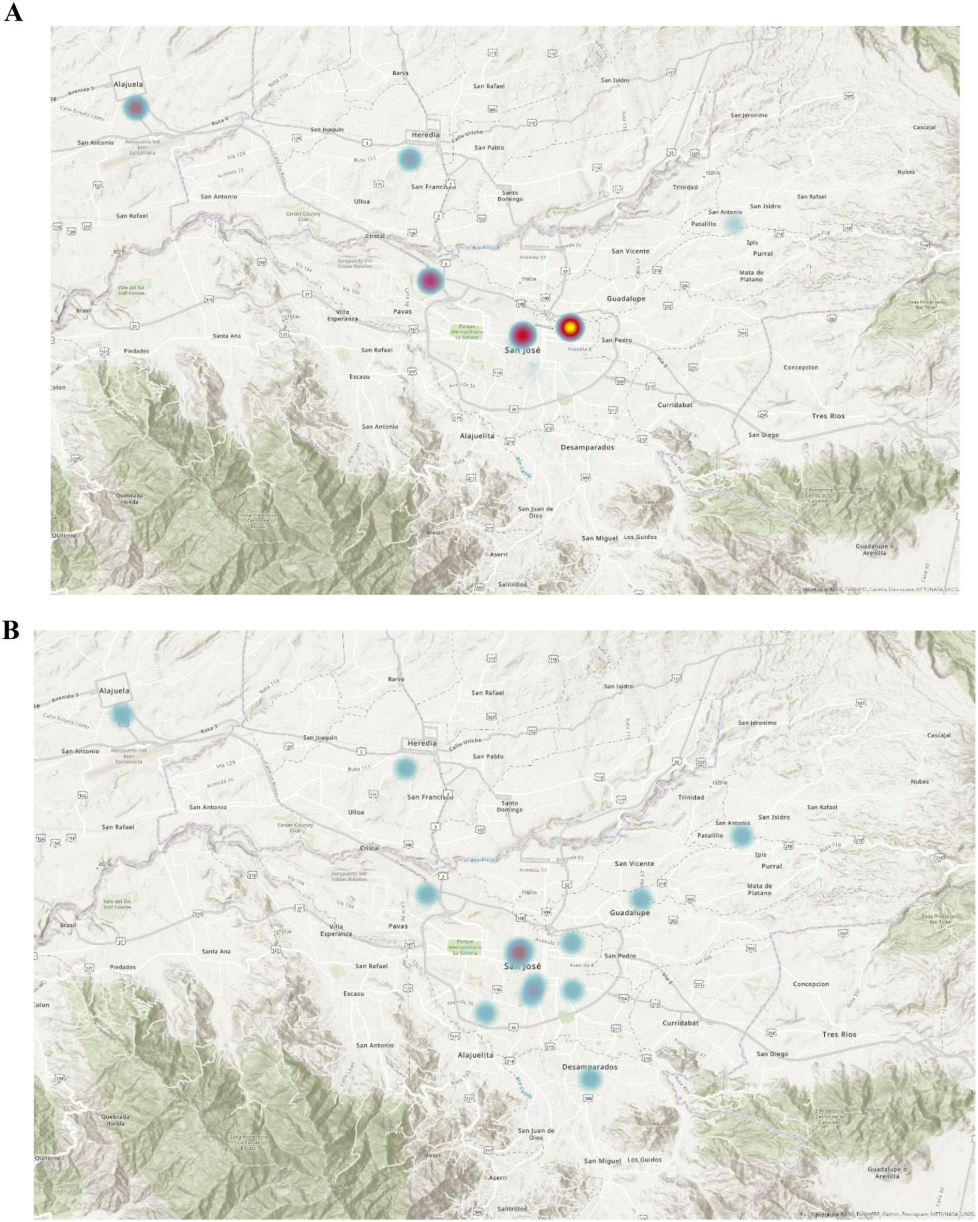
Heat map distribution of total ultrasound utilization (including point-of-care and consultative ultrasound) within Emergency Department and urgent care settings in 2006 and 2022 in the Greater San José Metropolitan Area. Denser utilization corresponds to warmer colors, while sparser utilization corresponds to cooler colors
